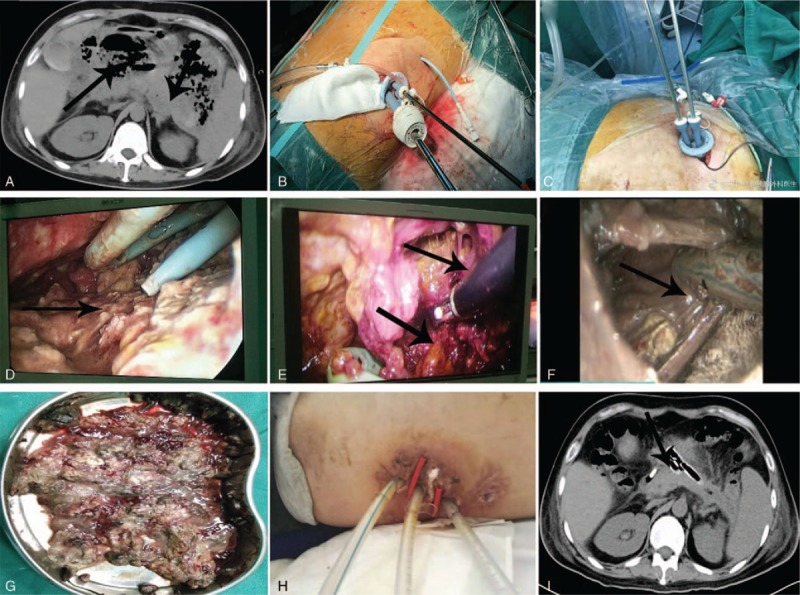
(A) Computed tomography before the first necrosectomy; (B, C, D, E, and F) single-incision access port retroperitoneoscopic debridement; (G) infected necrotic tissue; (H) minimally invasive incision; I: computed tomography after last necrosectomy.
